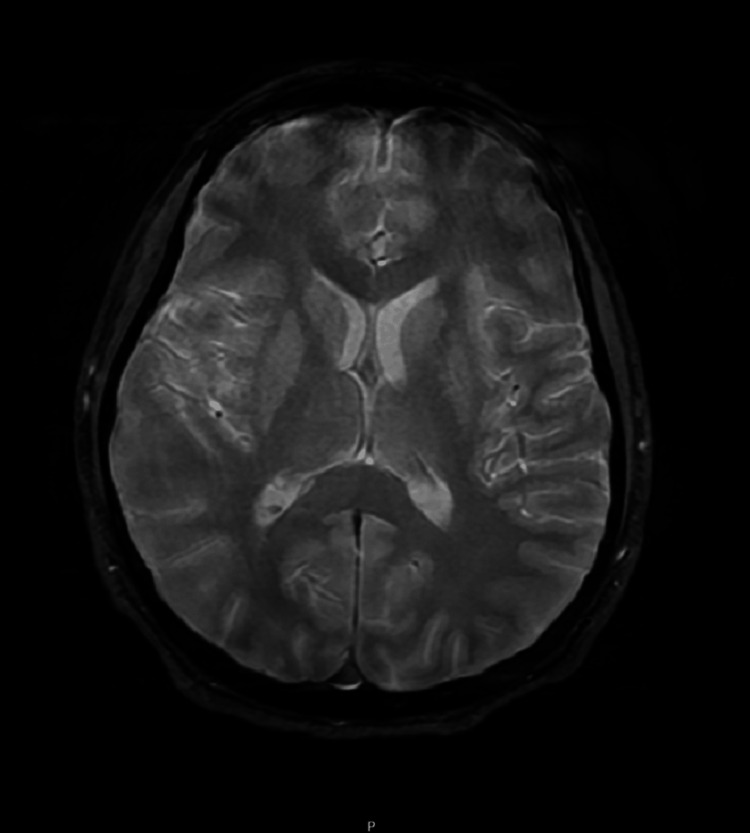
Axial T2-weighted brain MRI T2-weighted axial MRI demonstrating no acute abnormalities. Brain parenchyma appears structurally intact with normal ventricular size and no signs of edema, hemorrhage, or mass effect. Motion artifacts are noted, limiting sensitivity for subtle changes. MRI: magnetic resonance imaging
